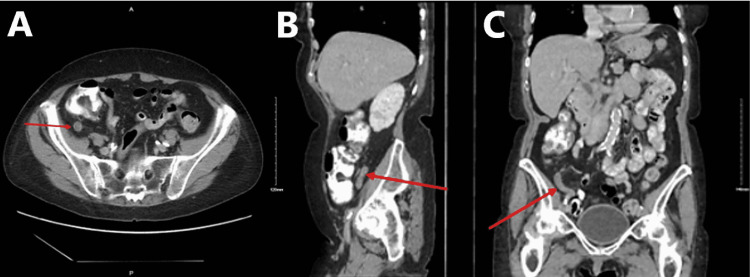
Computed tomography image of the abdomen and pelvis with the oral contrast demonstrating a thickened appendix of approximately 11 mm in the (A) transverse plane, (B) sagittal plane, and (C) coronal plane
